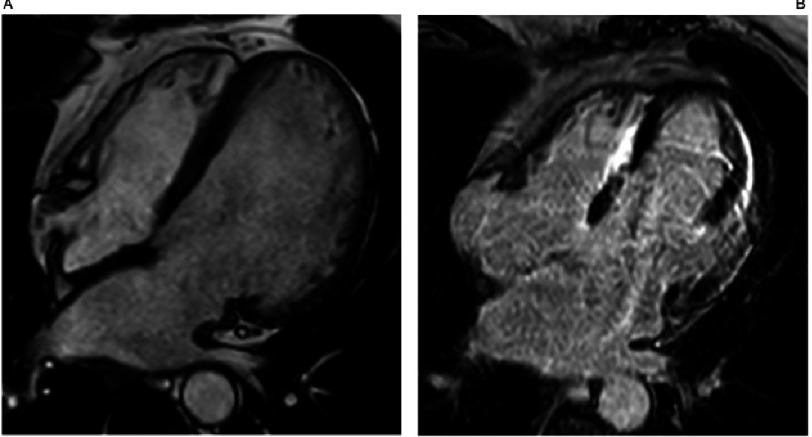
Cardiac Magnetic Resonance (CMR) Apical 4CH view: A) Cine and B) Late gadolinium enhancement images. End-stage HCM with progressive remodelling and sever systolic dysfunction, atrial fibrillation and ventricular tachycardia. MYH7 p.Arg453Cys.
